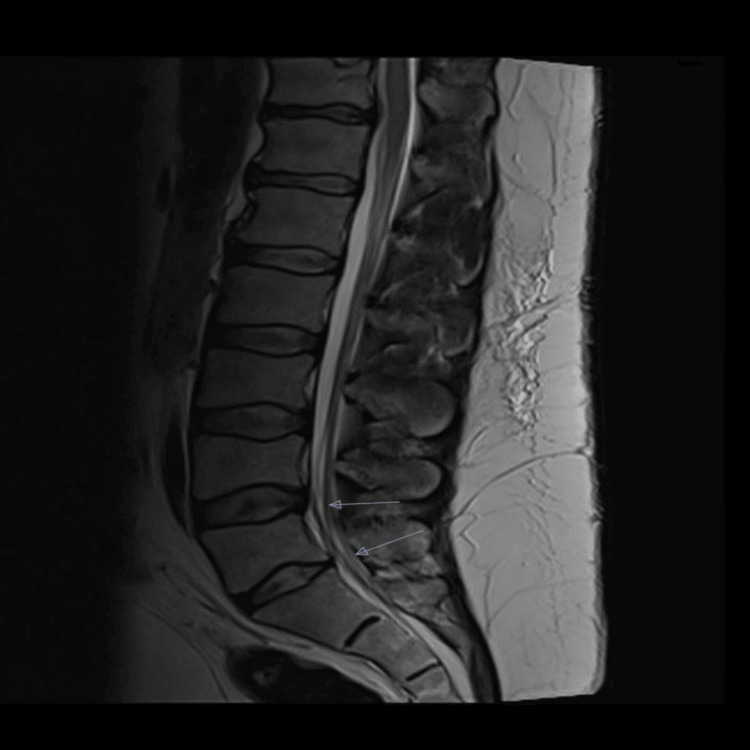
Sagittal T2-weighted MRI of the lumbosacral spine demonstrating spinal canal stenosis at the L4–L5 and L5–S1 levels. The blue arrows indicate the sites of stenosis.
